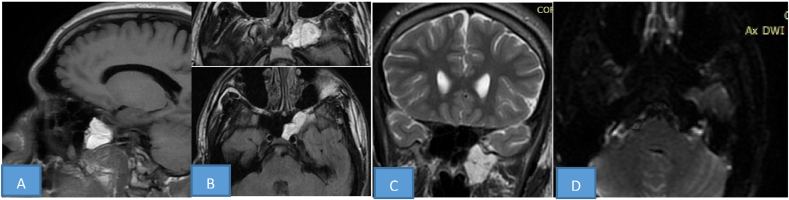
Brain RM image T1, T2 and DWI: Sagittal (A) and axial (B) T1 weighted (W) SE, and coronal(C) T2 axial (D) DWI: MR images show well defined lobulated non-expansive lesion located at the left sphenoid sinus pneumatization site (arrows). The lesion demonstrates increased signal on both T1W and T2W images which is consistent with fat signal.
